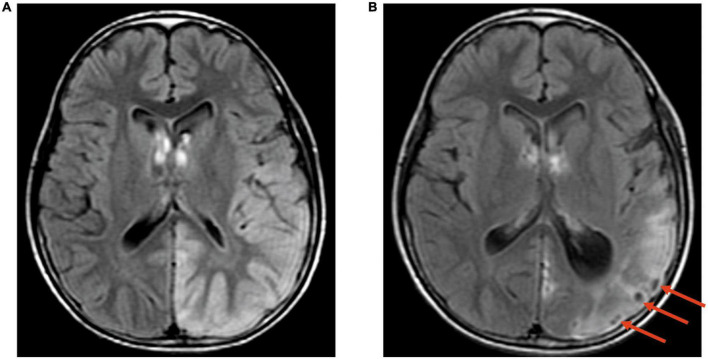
The black toenail sign. Case 12, A 12-year-old boy with MELAS. (A) Transverse FLAIR images. Hyperintensity in the left temporoparietal occipital lobe; (B) Transverse FLAIR images. The same patient was examined 3 months later again. Cerebral atrophy was observed in the left temporoparietal occipital lobe with the “black toenail” sign (shown by the red arrow) on the top of the gyrus.
